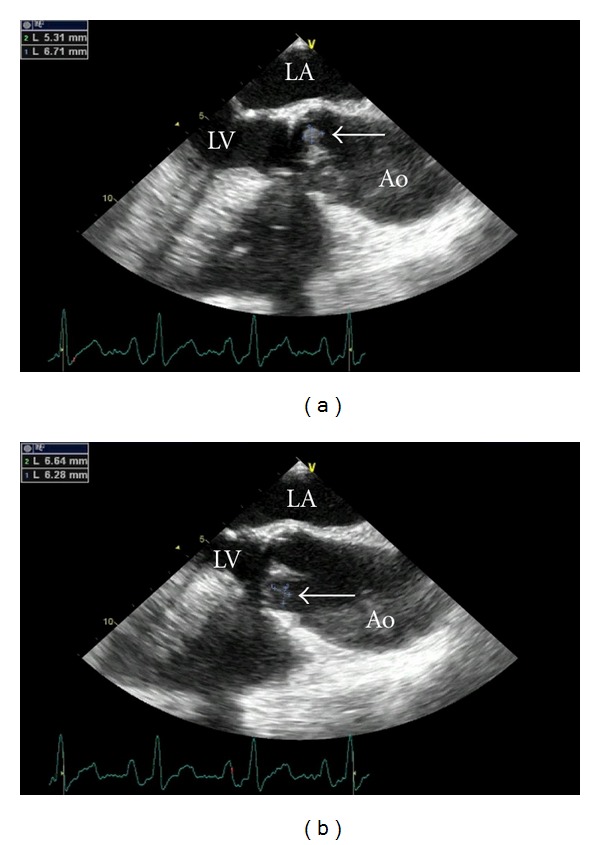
Transesophageal echocardiography. Echodense images on the aortic cups indicated by arrows. Left atrium (LA), left ventricle (LV), aorta (Ao).
